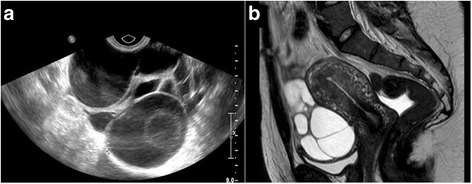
Multiple ovarian cysts revealed in pelvic ultrasonogaphy and MRI. Pelvic ultrasonography (a) revealed an enlarged 7x5 cm left ovary containing multiple cysts without fluid in the pelvis. This sagittal pelvic Magnetic Resonance Imaging (b) T2 weighted images showed a left multicystic ovary
